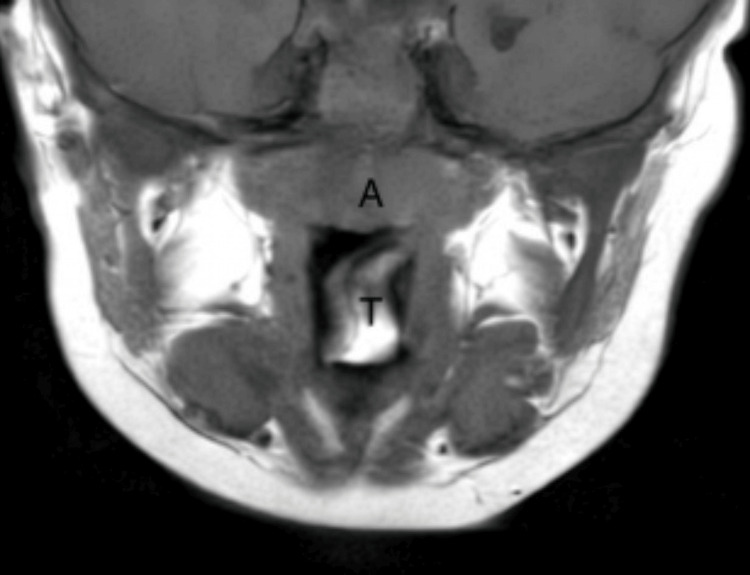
T1-weighted coronal MRI A: Adenoid; T: Teratoma.
